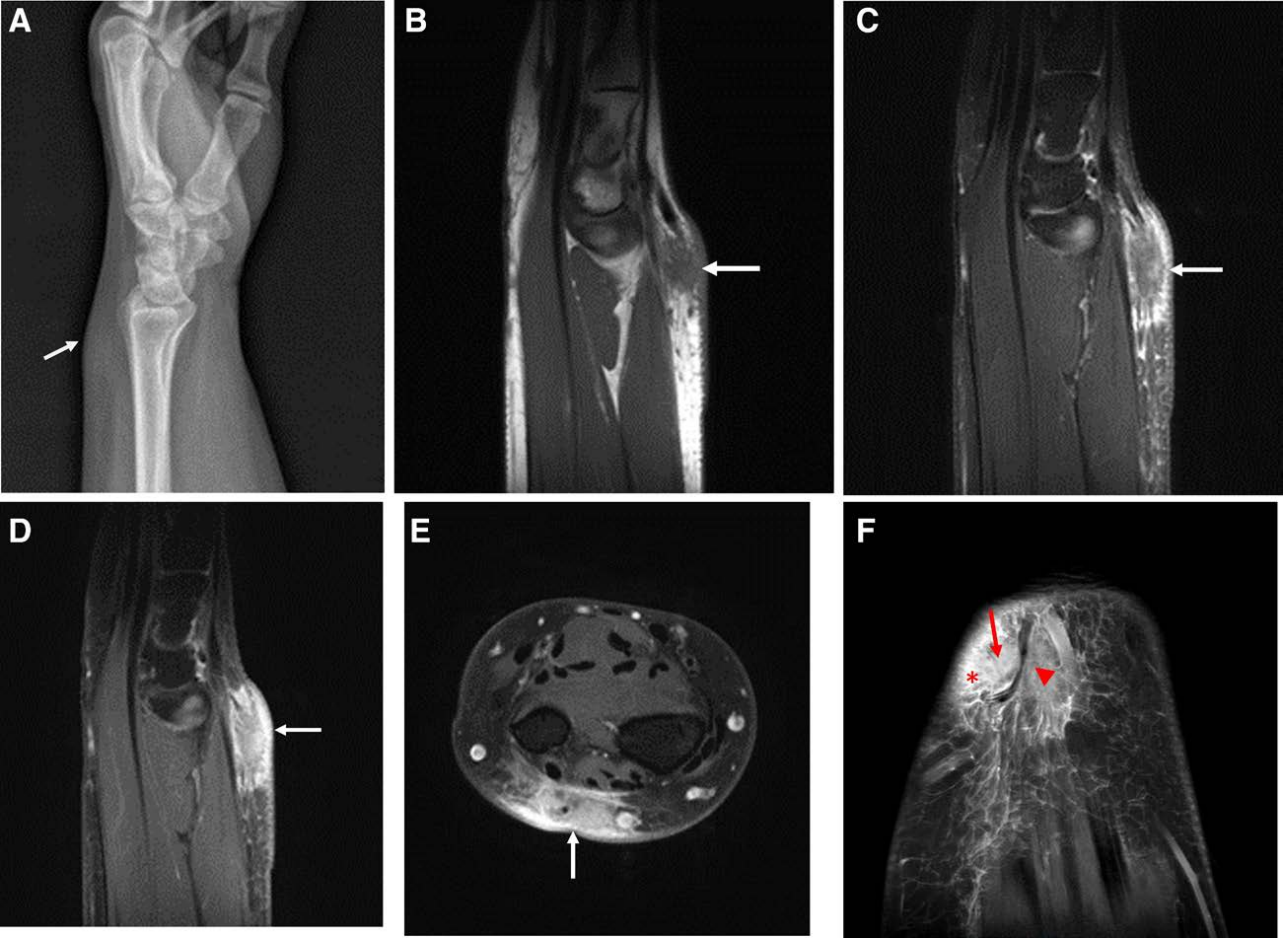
Plain lateral radiograph of the left wrist (A) shows a focal soft tissue swelling at the dorsal aspect (arrow). MRI of the wrist demonstrates an ill defined soft tissue mass (arrow) with low signal intensity (SI) on sagittal T1 weighted image (WI) (B) and intermediate SI on sagittal fat suppressed T2 WI (C). Postcontrast fat suppressed MR images (D–F) show ill defined, heterogeneously enhancement of the mass (arrows) with enhanced peritumoral edema (star in F) and neurovascular infiltrative appearance. However, vascular structures (arrowhead in F) in the enhanced mass appear intact. MRI = magnetic resonance imaging.
